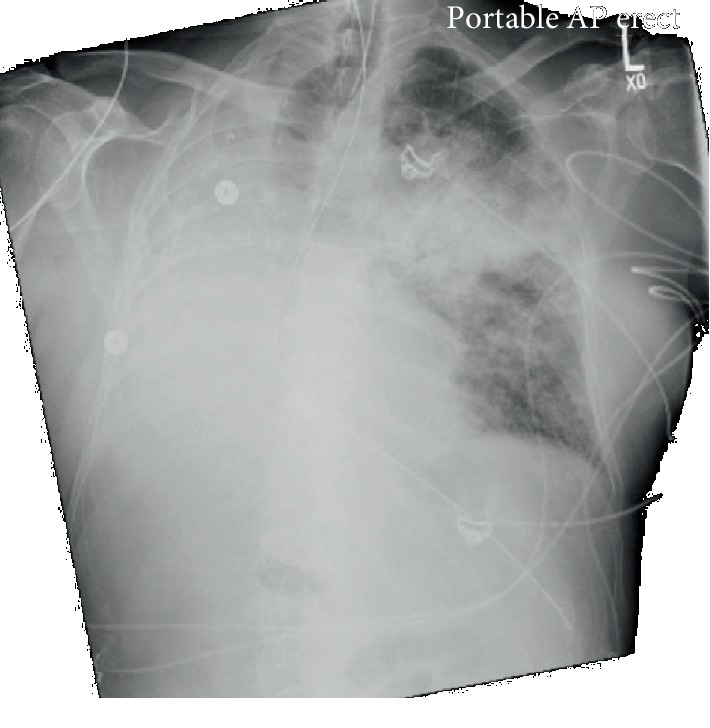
Repeat chest X-ray done one day after admission. Patient noted to have worsening hypoxia and increased work of breathing and was subsequently intubated. Chest X-ray significant for worsening of the left-sided superior perihilar infiltrate.
